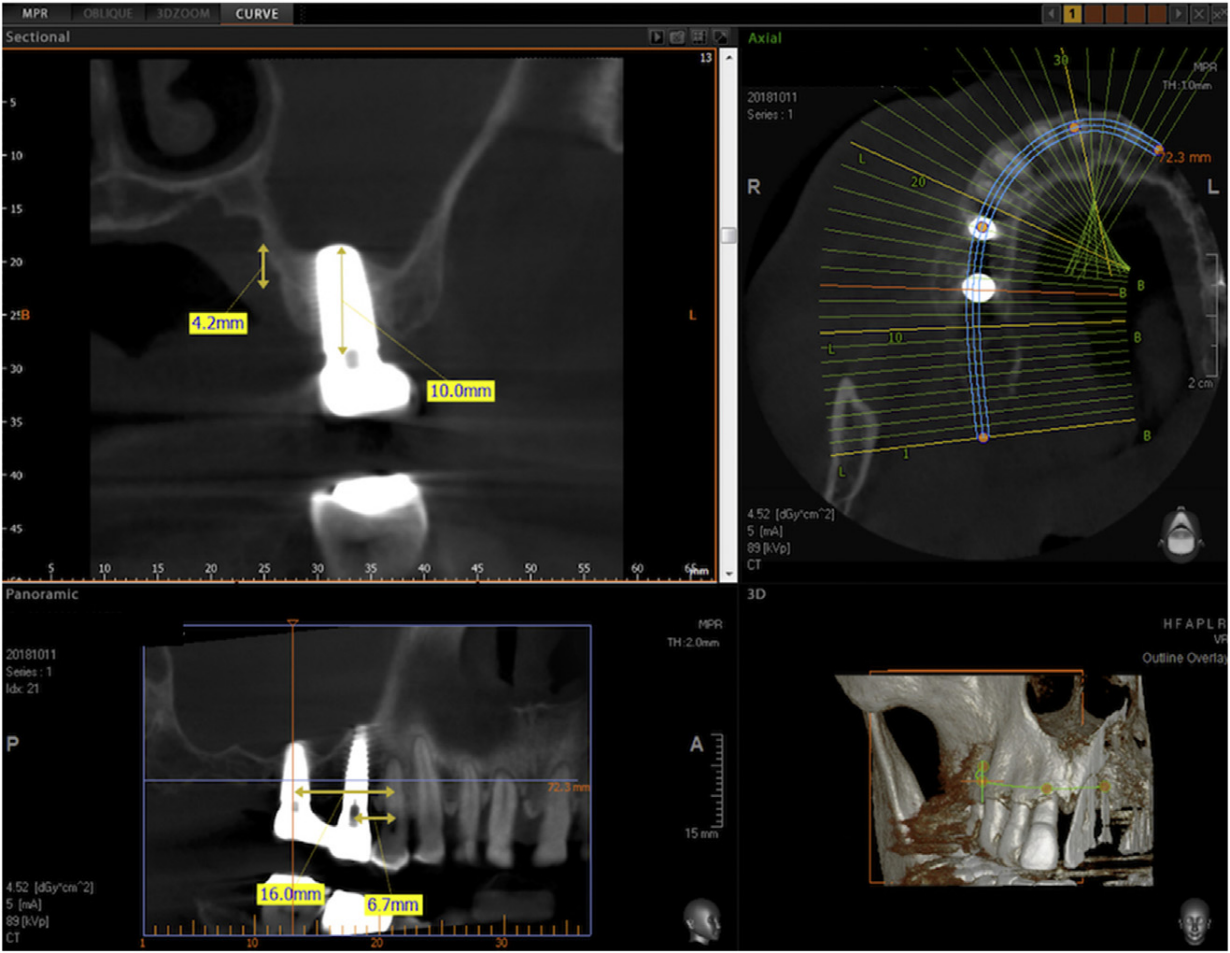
Detail of the CBCT of figure 12 centered on the implant in the first molar site. The implant is a 4.8 × 13 mm Sweden & Martina Global UXR. The apex of the implant is covered with newly formed bone. The vertical bone augmentation is about 4.2 mm.
